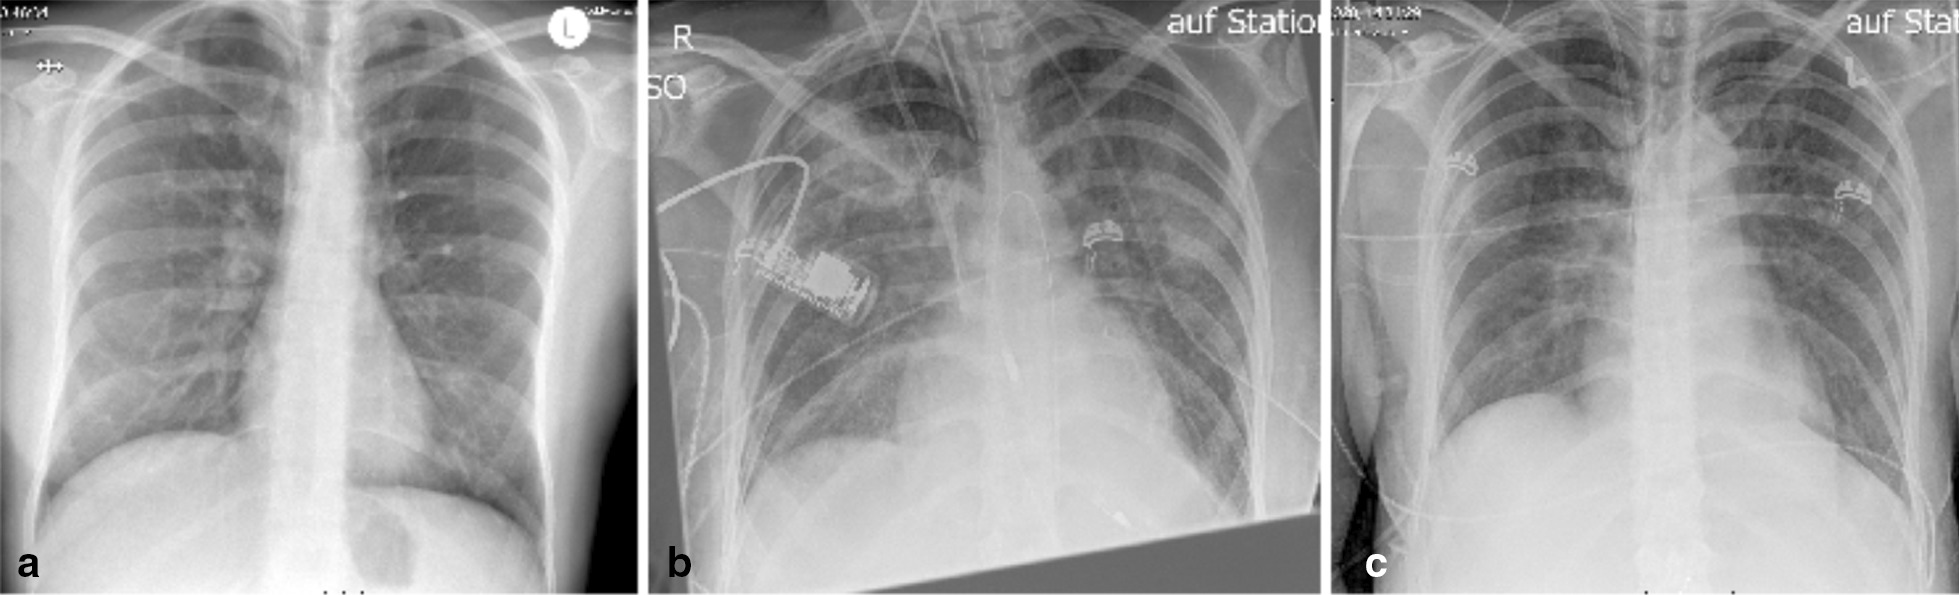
Chest radiography in supine position and anterior–posterior projection at admission (a), after Impella® placement (b) and at discharge (c). a Marked pulmonary congestion and mild bilateral pleural effusion. b Prominent bilateral pulmonary edema. c Residual pulmonary congestion
